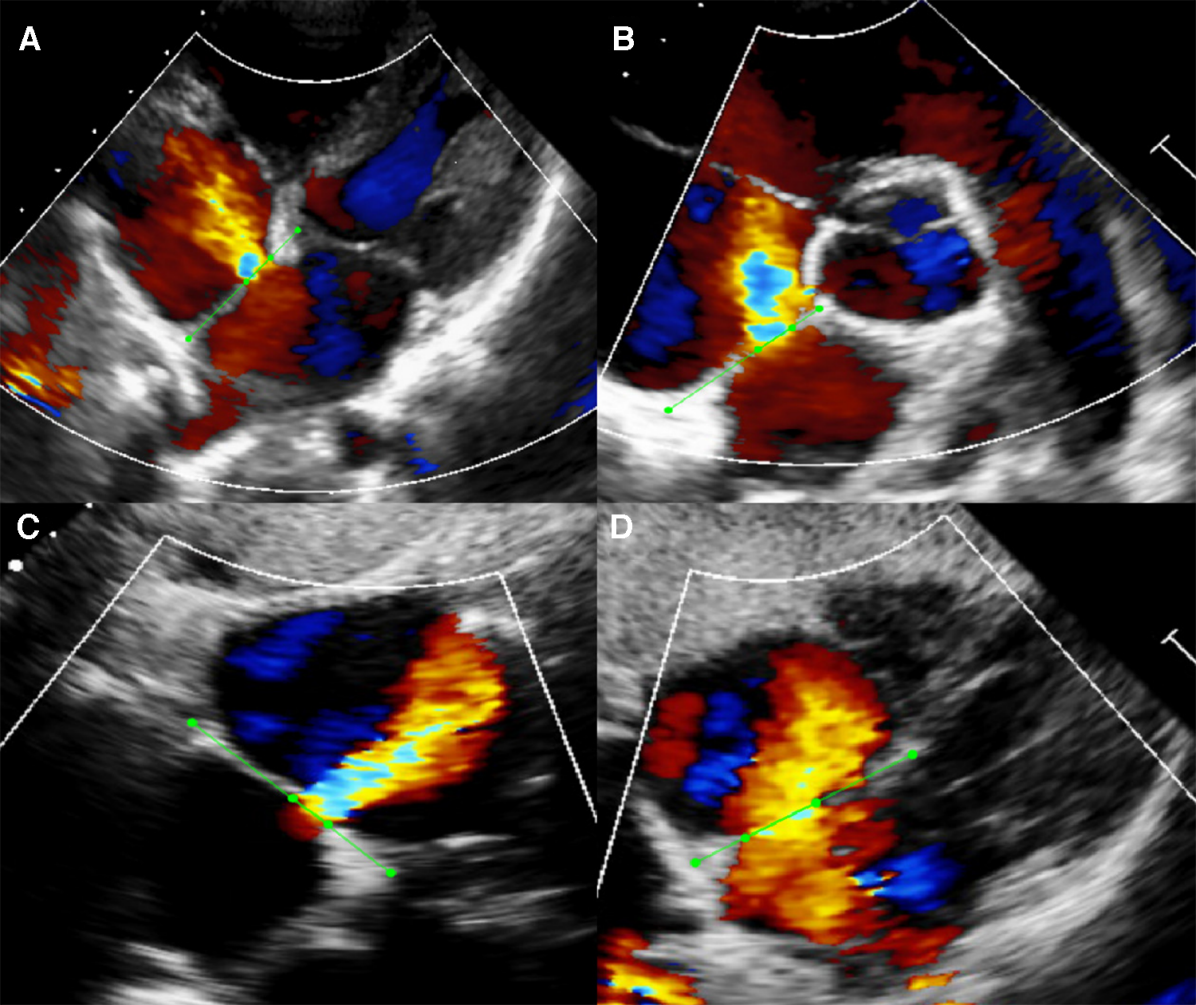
Example of manual segmentation. Green dots were manually labeled as the endpoints of atrial septum and defect with the open-source program LabelMe. (A) Modified apical four-chamber view (A4C). (B) Parasternal short-axis view (PSAX). (C) Subxiphoid sagittal view (SC2A). (D) Subxiphoid four-chamber view (SC4C).
